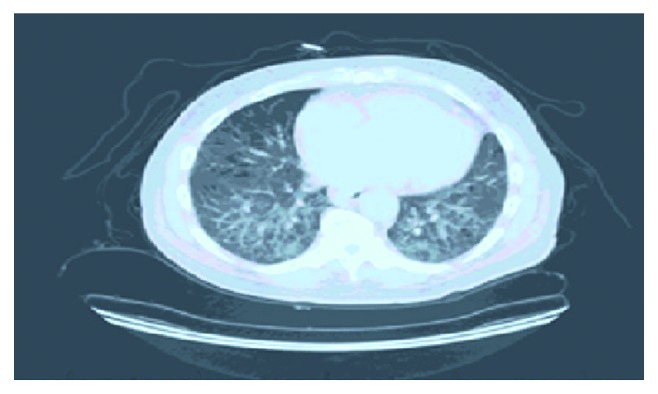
CT chest with contrast showing bilateral interlobular septal thickening, multiple ground-glass opacities, and cystic changes.
